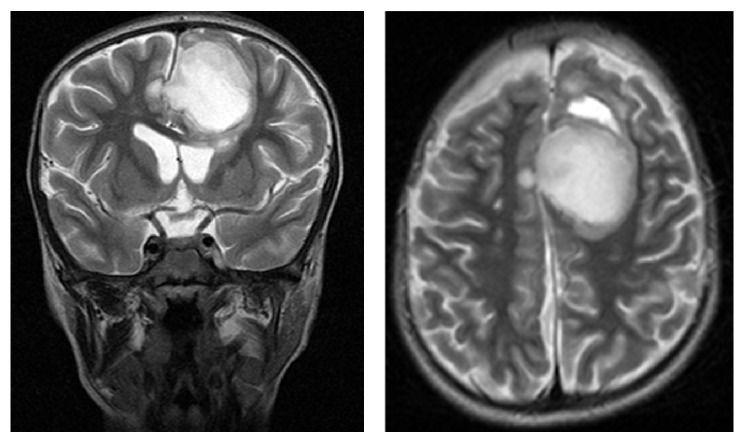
Brain MRI showing expansion of aspirated brain abscess to contralateral frontal lobe.
